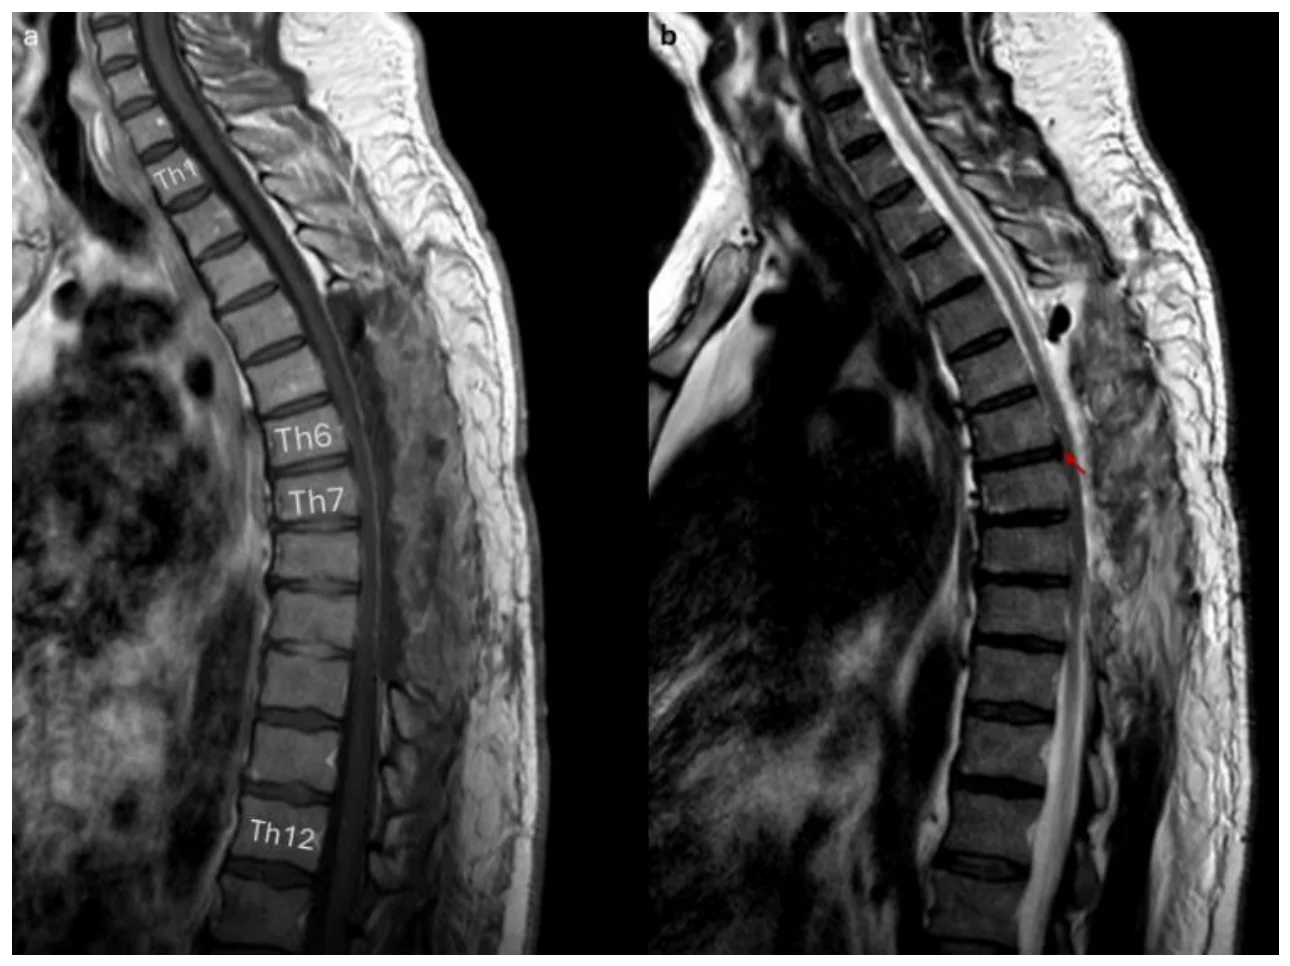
MRI of the thoracic region, post-operatively. Red arrow—Th6/Th7 intravertebral disc herniation. (a)—T1WI sequence, sagittal view; (b)—T2W1 sequence, sagittal view.
